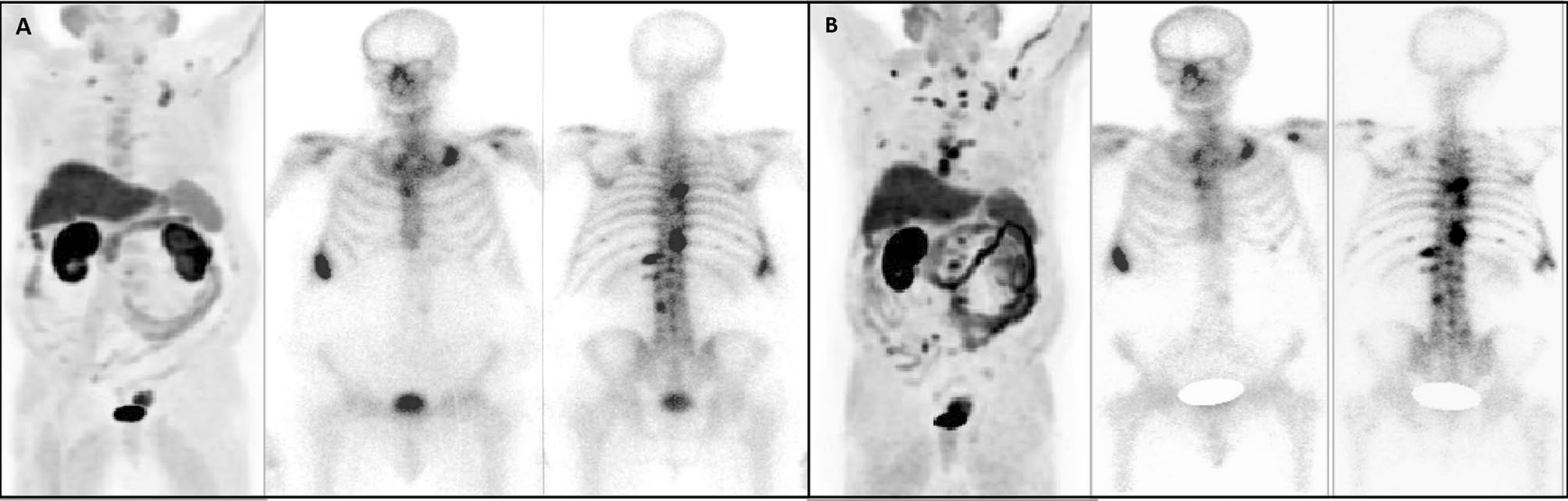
Patient ≠ 3. Baseline (A) and interim (B) series of FCH PET/CT (left images) and BS (central and right images) show polimetastatic osteoblastic disease. Interim BS was stable with respect to baseline study, whereas progression was detected on interim FCH PET/CT. 82 years old man, Gleason 9, ^223^Ra in third line, baseline PSA: 59 ng/ml. PFS of 3 months, under follow-up for OS.
